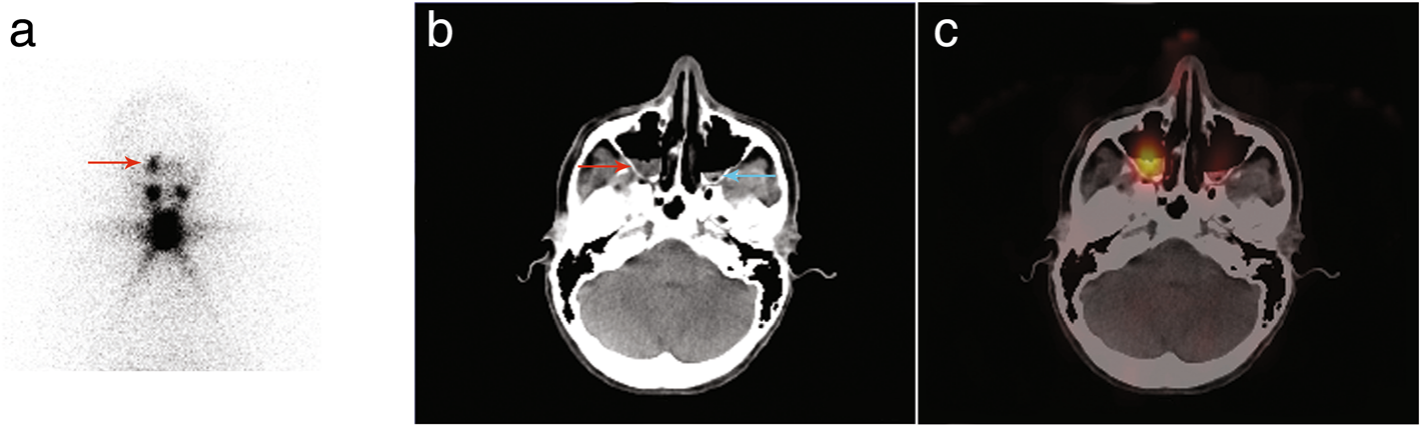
Planar acquisition (anterior view, Panel a), CT (Panel b) and fused (Panel c) images of SPECT/CT acquisition displaying RAI uptake in both the right maxillary sinus (*red arrow*) and the left one (*blue arrow*) with bilateral mucosal thickening on CT scan slices
